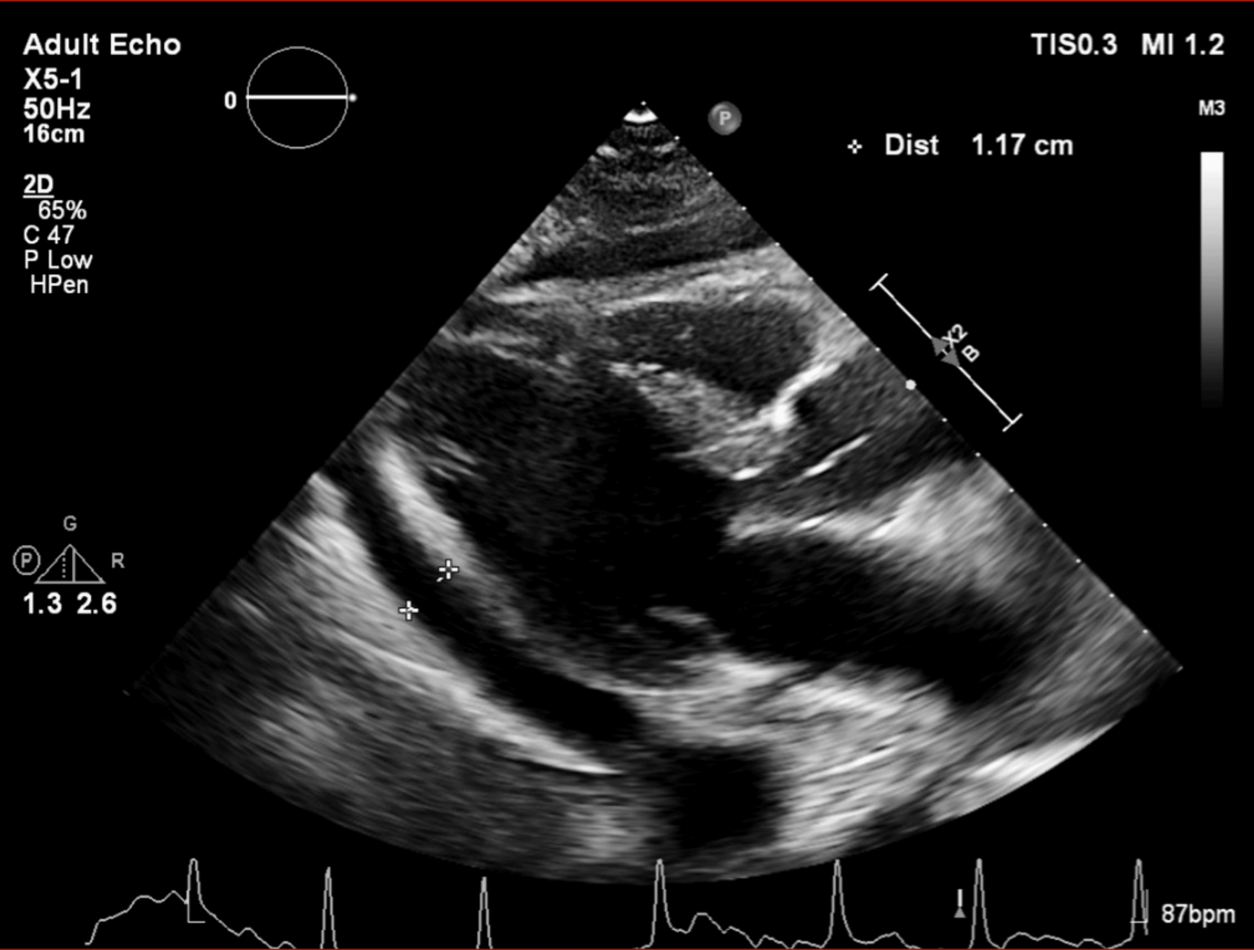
Transthoracic echocardiogram (parasternal long-axis view) showing pericardial effusion The measurement markers demonstrate a pericardial fluid collection of approximately 1.17 cm.
